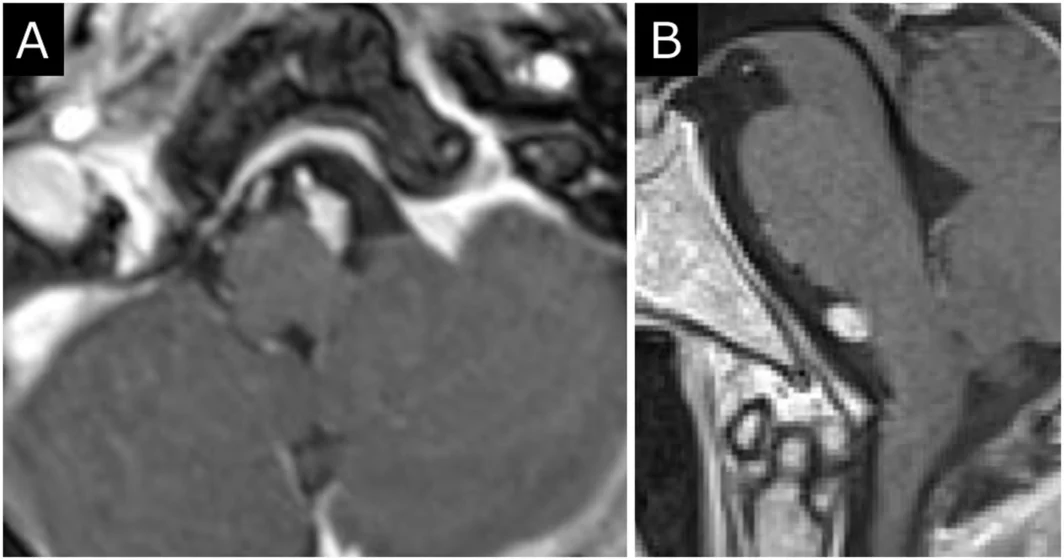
(A, B) Postoperative MRI shows residual tumor on the ventral surface of the medulla oblongata. MRI: Magnetic resonance imaging.
